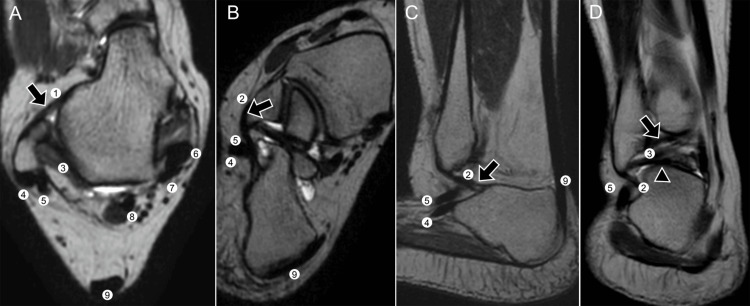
Lateral ligament complex on volumetric T2-weighted CUBE MRI. Multiplanar reconstructions depict (1) anterior talofibular ligament (ATFL), (2) calcaneofibular ligament (CFL), (3) posterior talofibular ligament (PTFL), (4) peroneus longus tendon, (5) peroneus brevis tendon, (6) posterior tibial tendon, (7) flexor digitorum longus tendon, (8) flexor hallucis longus (FHL) tendon, and (9) calcaneal (Achilles) tendon. Three-dimensional views show (A) ATFL (arrow); (B, C) CFL (arrow); (D) PTFL including deep fibers (arrow) and superficial fibers (arrowhead). Images belong to the authors; patient consent was obtained.
